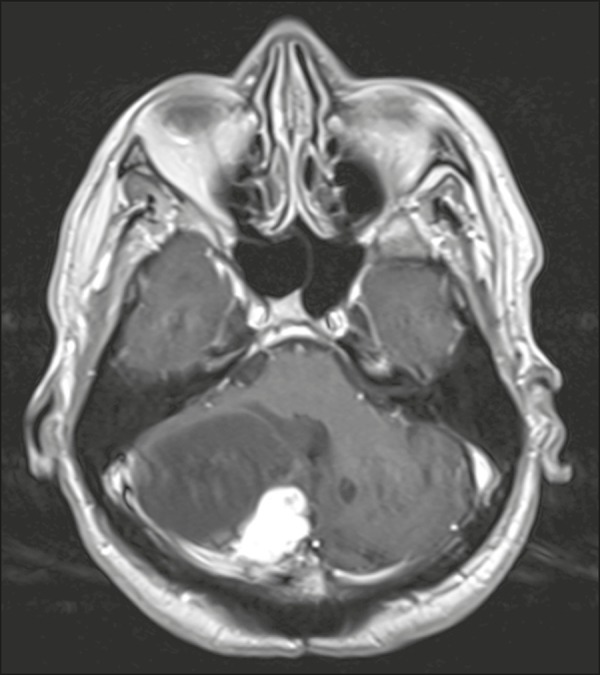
Hemangioblastoma. Contrast-enhanced axial T1-weighted MRI
